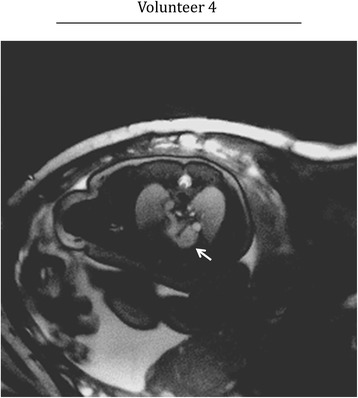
Visualization of congenital heart defect using the current approach. An abnormal three vessel view is shown wherein the ascending aorta (arrow) is larger than the main pulmonary artery. This abnormality indicates tetralogy of Fallot which was confirmed by ultrasound. A cine corresponding to this reconstruction is available as supporting video S6
